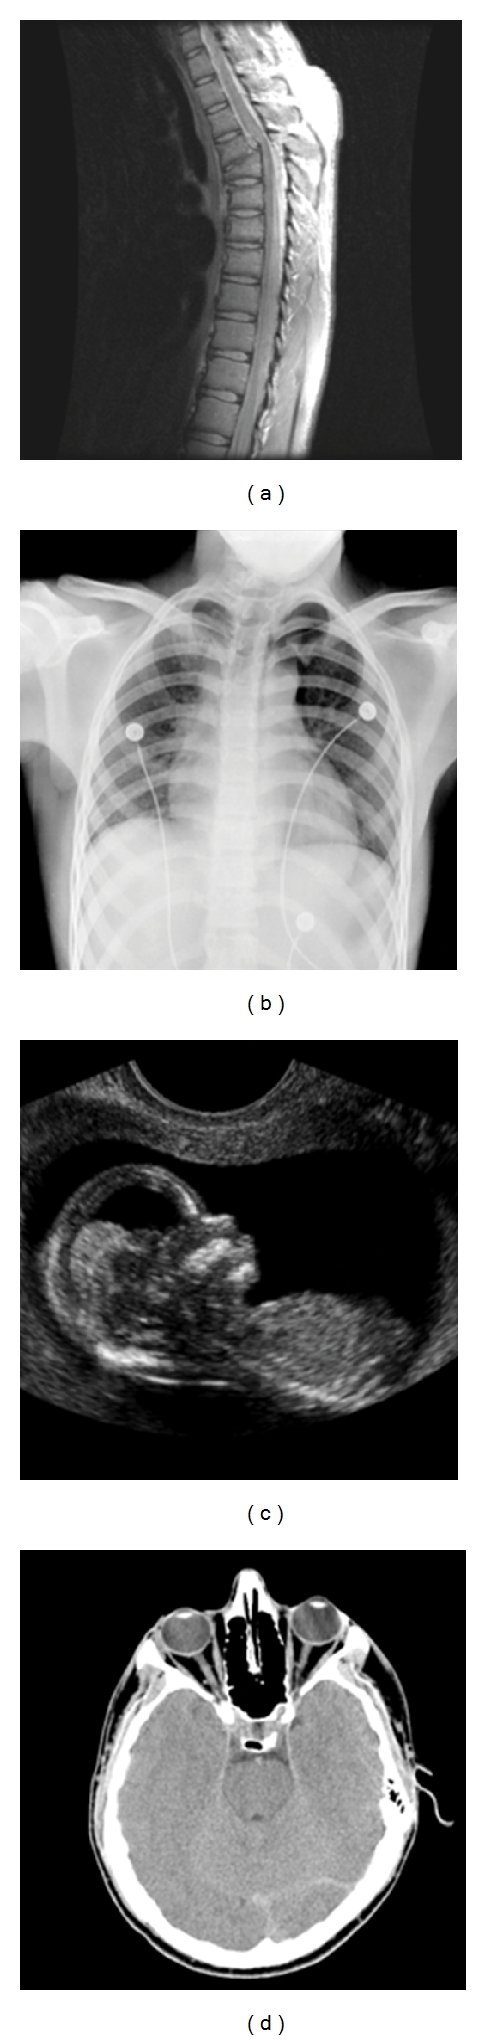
The 256 × 256 host images with 256 grey levels; (a) spine (MRI), (b) chest (X-ray), (c) fetus (ultrasonic), and (d) head (CT).
